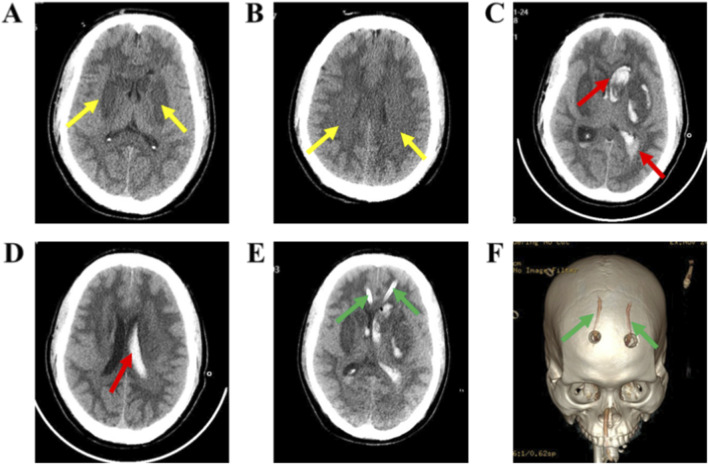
Dynamic changes on cranial CT during treatment. Initial non-contrast CT on admission (A,B): (A) Axial non-contrast CT shows symmetric hypoattenuation in the bilateral basal ganglia, predominantly involving the lentiform nuclei (yellow arrows). (B) Axial non-contrast CT shows patchy hypoattenuation in the deep white matter of both cerebral hemispheres at the level of the corona radiata/centrum semiovale (yellow arrows). Follow-up CT after clinical deterioration (C,D): (C) Axial non-contrast CT demonstrates an acute intracerebral hematoma in the left basal ganglia (predominantly the lentiform nucleus) with intraventricular extension and associated intraventricular hemorrhage; marked mass effect is present, including ventricular compression, midline shift, and effacement of sulci and basal cisterns, indicating a risk of herniation. (D) Repeat axial non-contrast CT shows interval evolution of the left basal ganglia hematoma and intraventricular hemorrhage (red arrow), with a corresponding change in mass effect. Postoperative CT (E,F): (E) Postoperative axial non-contrast CT shows bilateral frontal external ventricular drains (EVDs; green arrows) with catheter tips positioned in the frontal horns of the lateral ventricles; residual intraventricular hemorrhage persists. (F) Three-dimensional CT reconstruction depicts the bilateral frontal burr holes/catheter entry sites (green arrows), corresponding to the surgical drainage tract.
